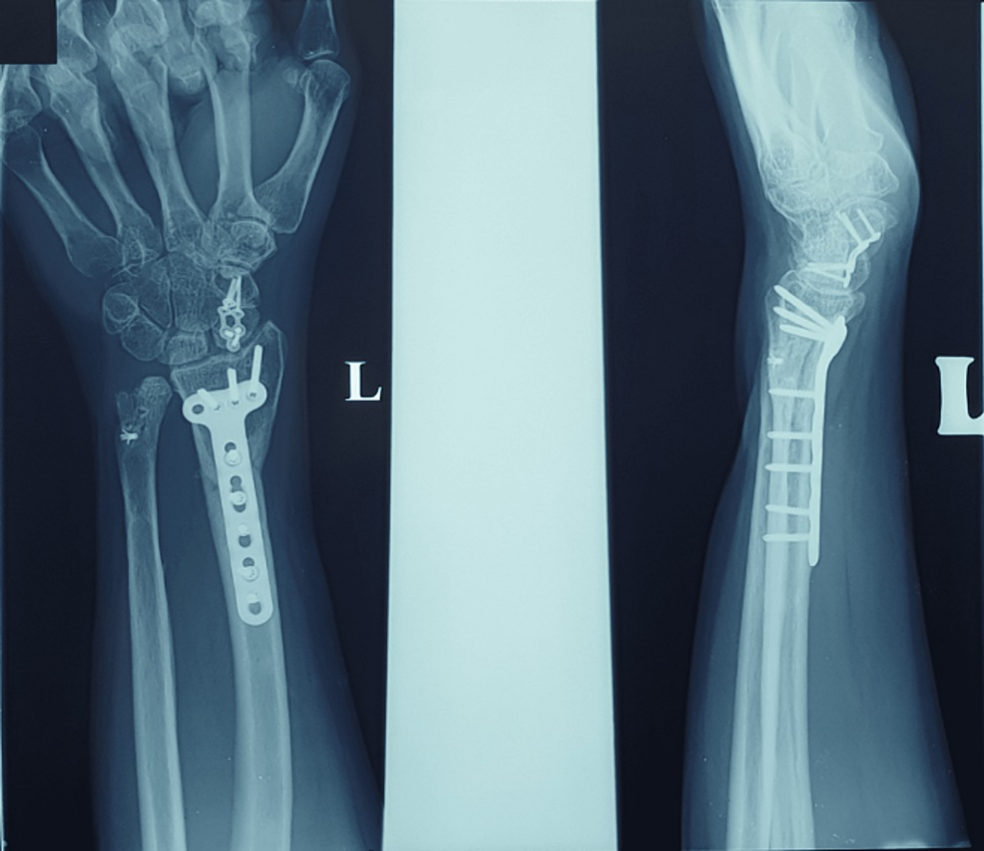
Radiographs showed hardware prominent and impingement of the proximal plate on the volar lip of the radius
